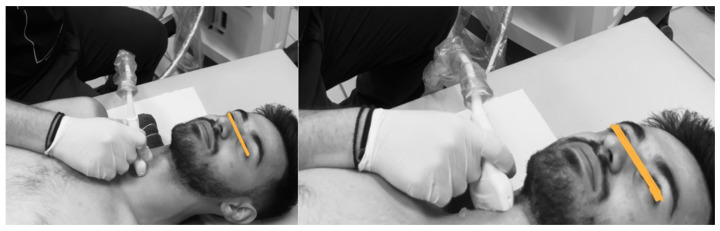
Ultrasonography of the anterior neck muscles (Longus Colli—left. Sternocleidomastoid—right).
